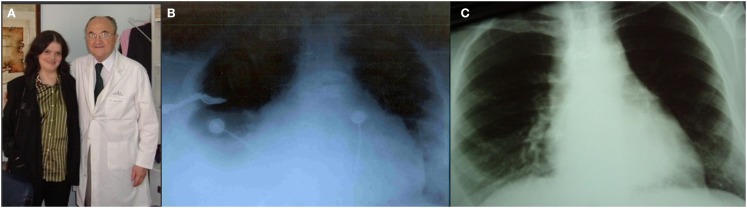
(A) Patient aged 53 years with TA Ib. After 34 years of Fontan Kreutzer palliation. (B) Pre-reconversion chest Roentgenogram. (C) Chest Roentgenogram 1 year after conversion.
